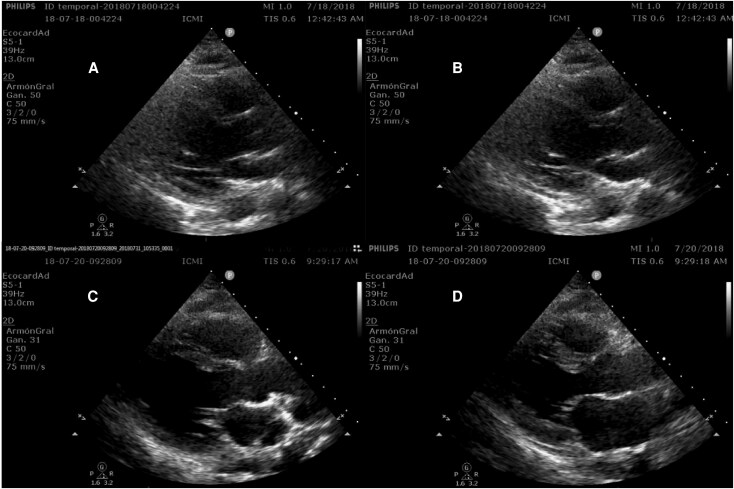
Graphical representation of TTS evolution (Patient 1). Transthoracic echocardiogram with a parasternal view in the acute phase demonstrating severe left ventricular dysfunction (LVFS 10%), ventricular diastole (*A*) and systole (*B*), showing akinesia of the mid and apical segments of the septum and lateral wall. In the recovery phase, improvement of left ventricular function (LVFS 45%) is demonstrated within the following 72 h, diastole (*C*) and systole (*D*).
